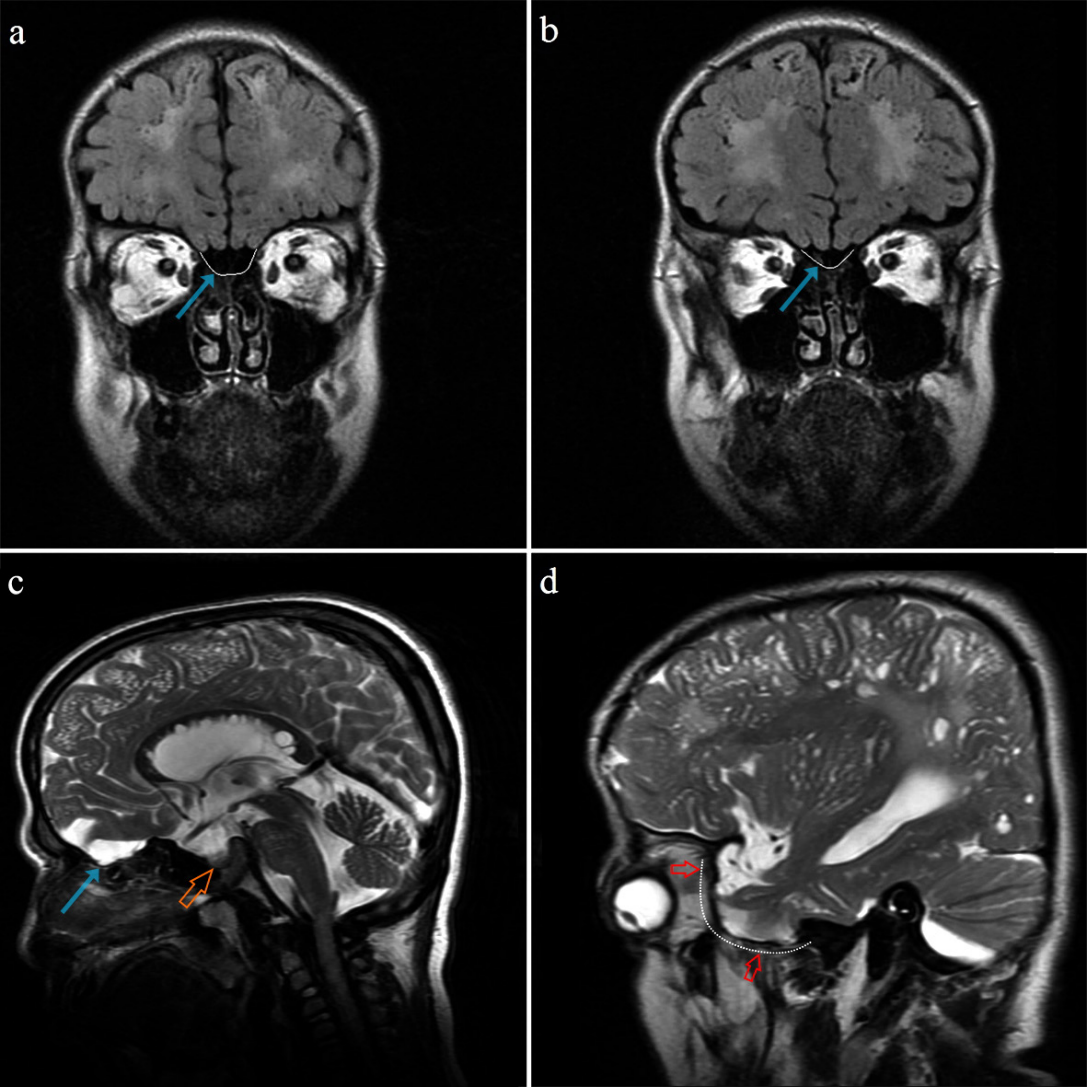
Coronal FLAIR (a, b) and sagittal T2 weighted (c, d) MR images demonstrate concave remodeling of ethmoid roof (blue arrows) and greater wing of the sphenoid bone (red arrows). There is also a ‘J shaped sella’ form (on image c) shown with an orange arrow (15 years old, MPS II).
